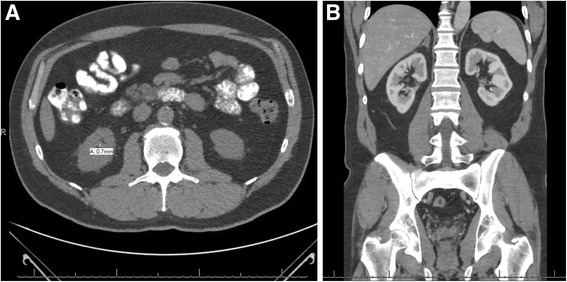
a Four-month follow-up computed tomography scan. Improving appearance of right adrenal gland with fluid measuring maximally 1.2 cm transverse diameter and no other identifiable lesions. Periadrenal fatty stranding was completely resolved. b Coronal view at 4-month follow-up
